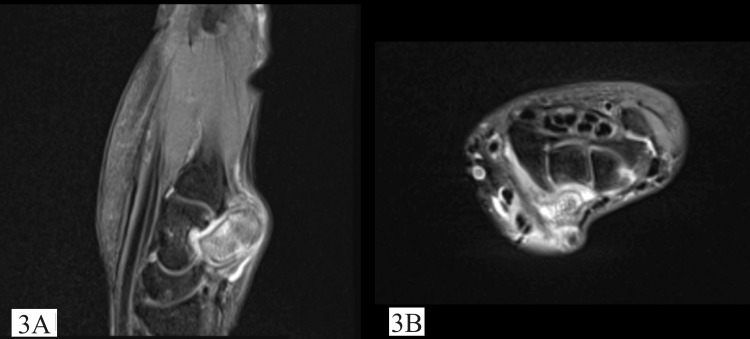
Pre-operative sagittal (A) and axial (B) magnetic resonance images depicting a left wrist bony lesion arising at the level of the lunate and triquetral proximally and capitate distally with increased signal intensity on the T2-weighted image (T2WI) image sequence
